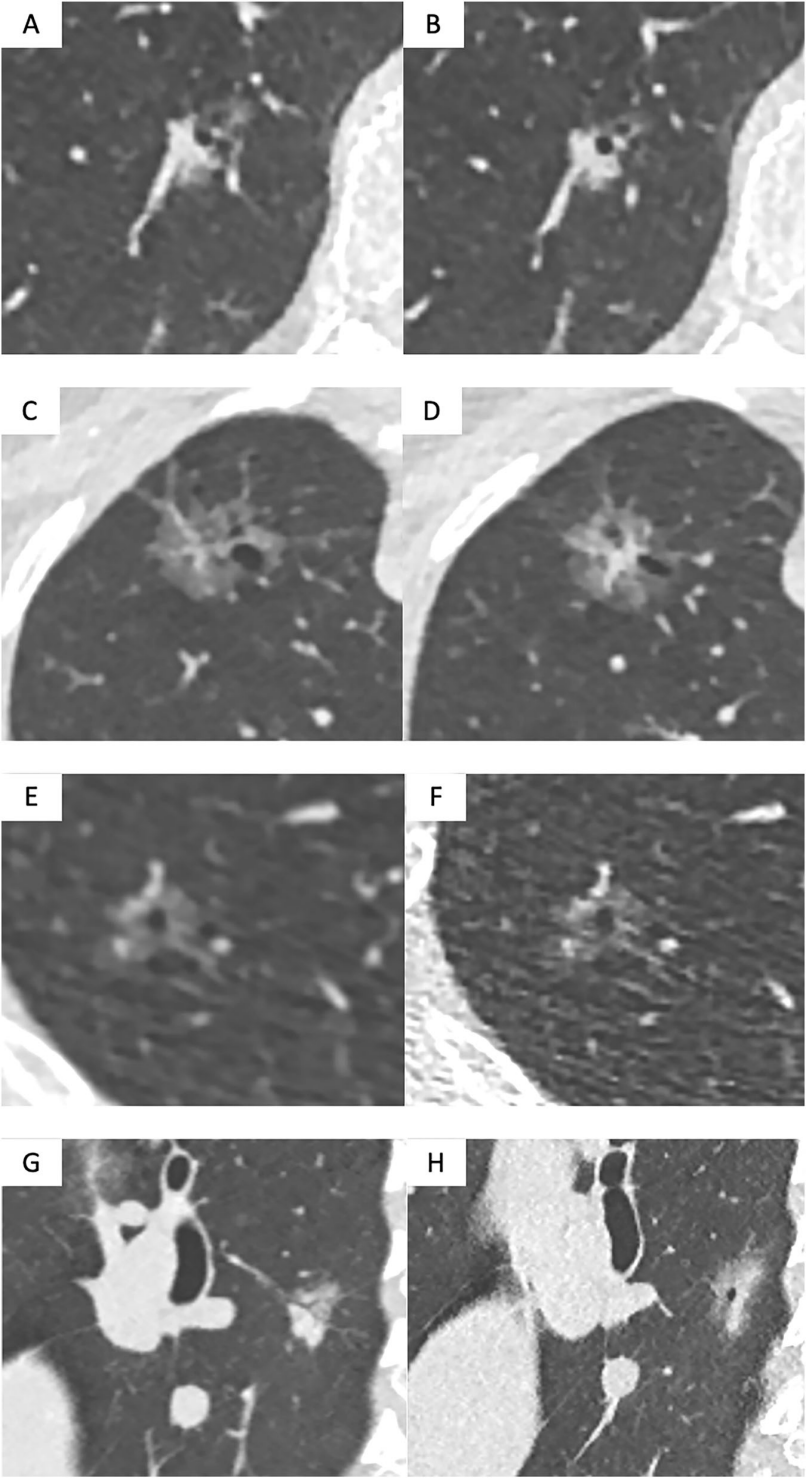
Suspicious morphological signs of ‘bubble-like lucencies’ and/or ‘air bronchogram’ in four patients. A, B Low-dose axial CT images showing a 17-mm part-solid nodule of the right lower lobe with an anterior ground-glass component (A) and bubble-like lucencies (A, B), in a 69-year-old woman who smoked 50 pack-years. Since the nodule has a solid component that is larger than 80% of the whole nodule, according to the ESTI guidelines, it would be managed as a solid nodule. A pT1bN0R0 invasive adenocarcinoma was confirmed postoperatively. C, D Low-dose axial CT images showing a part-solid nodule of the right upper lobe with a 7-mm solid component and bubble-like lucencies in a 64-year-old woman who smoked 35 pack-years. A pT1cN0R0 invasive adenocarcinoma was confirmed postoperatively. E, F Low-dose axial CT images in soft kernel (E) and high frequency kernel (F) showing a part-solid nodule of the right upper lobe with small non-measurable solid components and central bubble-like lucency in a 54-year-old woman with a smoking history of 35 pack-years. The high-frequency kernel image (F) is noisier. A pT1bN0R0 invasive adenocarcinoma was confirmed postoperatively. G, H Low-dose sagittal CT images showing a 24-mm part-solid nodule of the right lower lobe with an air bronchogram (G) and bubble-like lucency (H), in a 52-year-old woman with a smoking history of 30 pack-years. A pT1bN0R0 invasive adenocarcinoma was confirmed postoperatively
